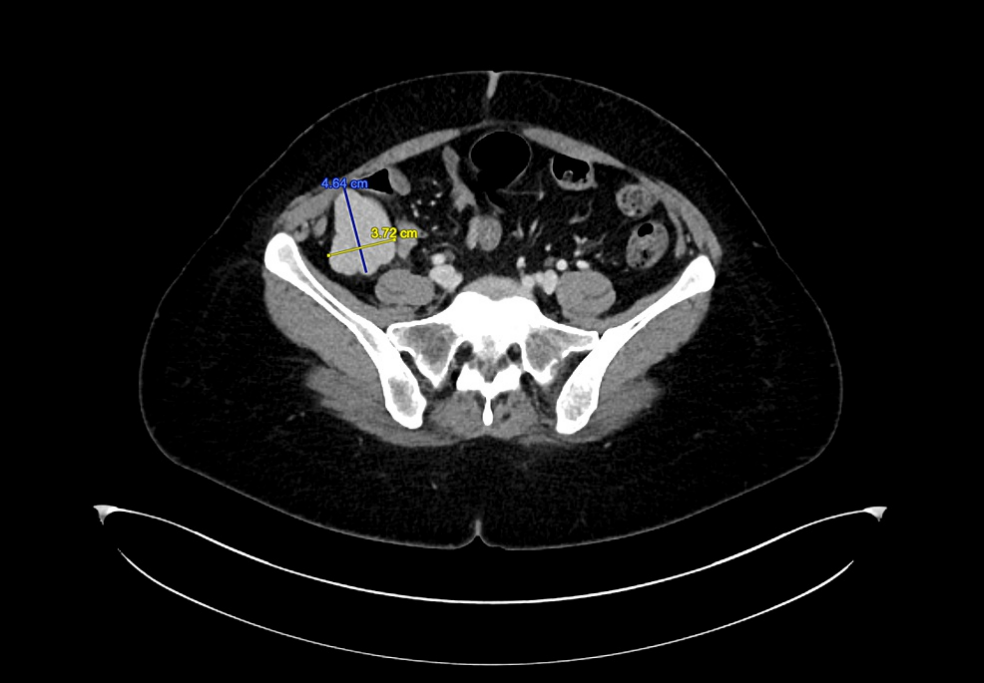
Axial CT scan of the abdomen and pelvis showing right lower quadrant enhancing mass with internal calcification, intense enhancement of the capsule due to paten vascular supply in the spleen’s capsule.
